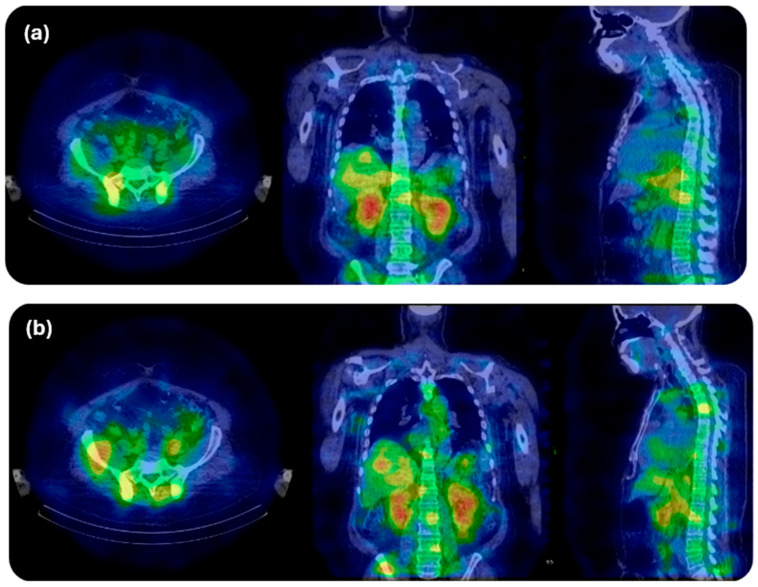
SPECT/CT feasibility for clinical image activity quantification of ^225^Ac-derived emissions. (a) Acquisition using the 218 keV photon emission, arising primarily from the decay of ^217^Fr. (b) Acquisition using the 440 keV photon emission, originating predominantly from the decay of ^213^Bi.
